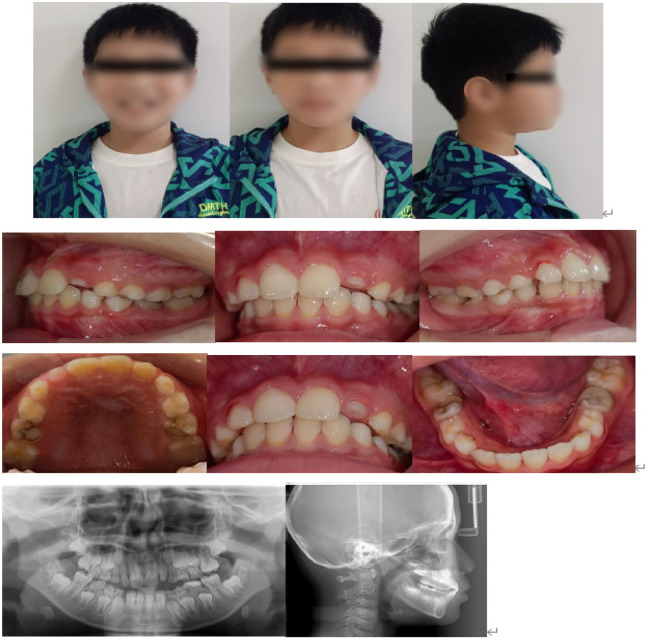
Post-treatment facial view, intraoral view, and x-ray film.
